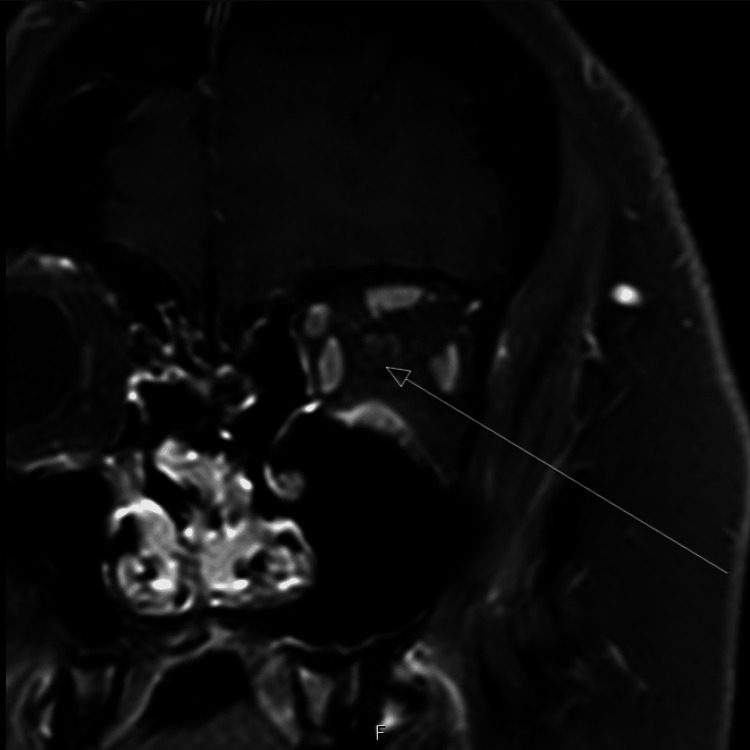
Coronal T1-weighted fat-saturated post-contrast MRI of the orbits obtained during follow-up, demonstrating complete resolution of previously noted left optic nerve enhancement (arrow), indicating response to immunosuppressive therapy and disease stability.
